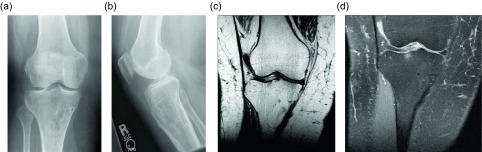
Intraosseous lipoma: 61-year-old female with chronic left knee pain. Frontal (a) and lateral (b) radiographs of the asymptomatic right knee obtained for comparison. Radiographs demonstrate a lucent lesion in the right tibial metaphysis with sclerotic margins and a narrow zone of transition. There is no periosteal reaction or associated soft tissue mass. Coronal T1W (c) and PD FS (d) images show the tibial metadiaphyseal lesion as isointense to fat on T1W with signal loss on the proton density fat saturated image.
